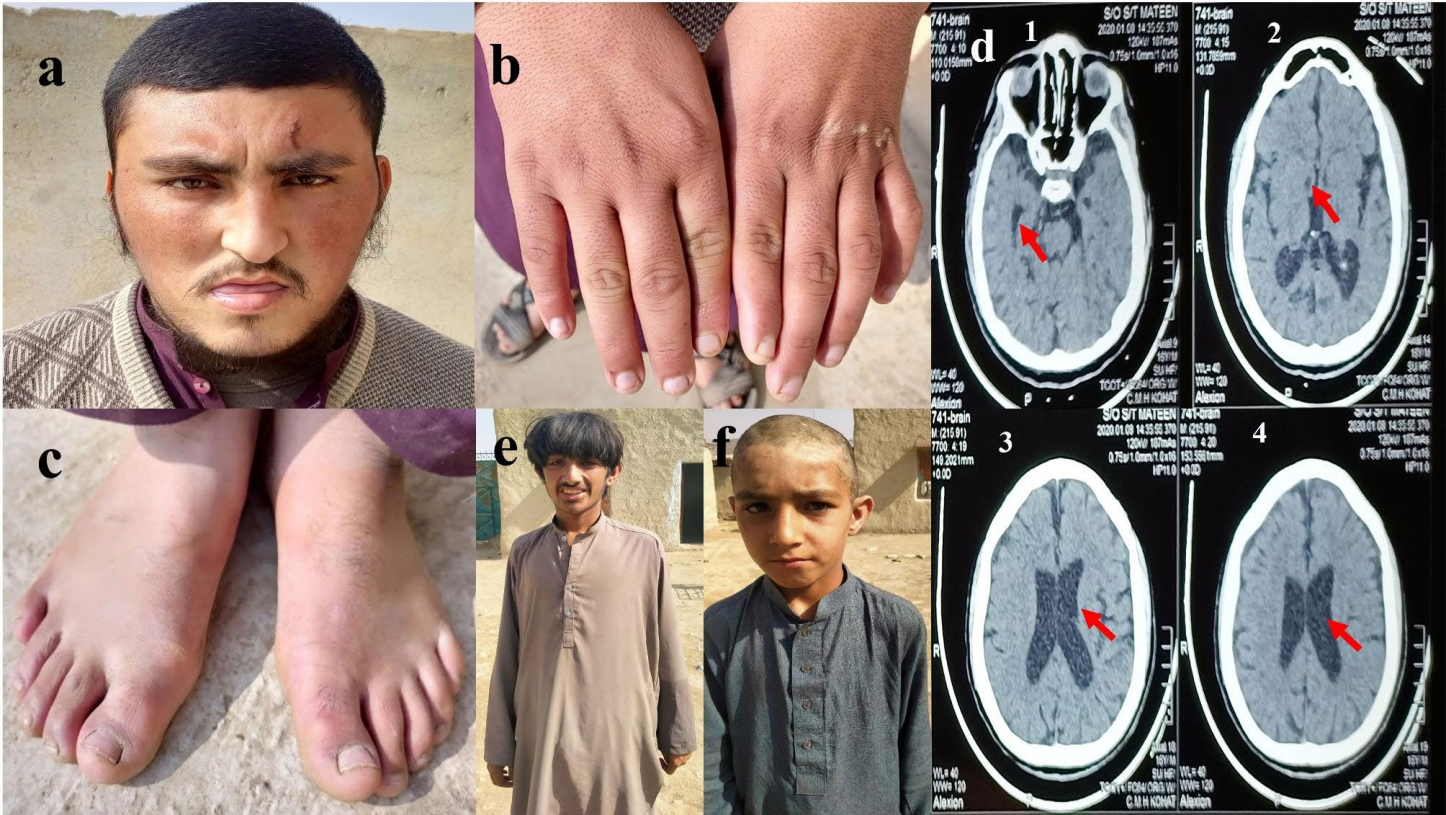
(a, b, c) Physical features presented by affected individual (V-2) of family A and (d) Computed tomography (CT) scan image of the affected individual (V-2) of family A indicative of multiple large CSF spaces within and around the brain suggestive of cerebral atrophy, (e, f) physical features presented by affected individuals (V-3 and V-4) of family B
